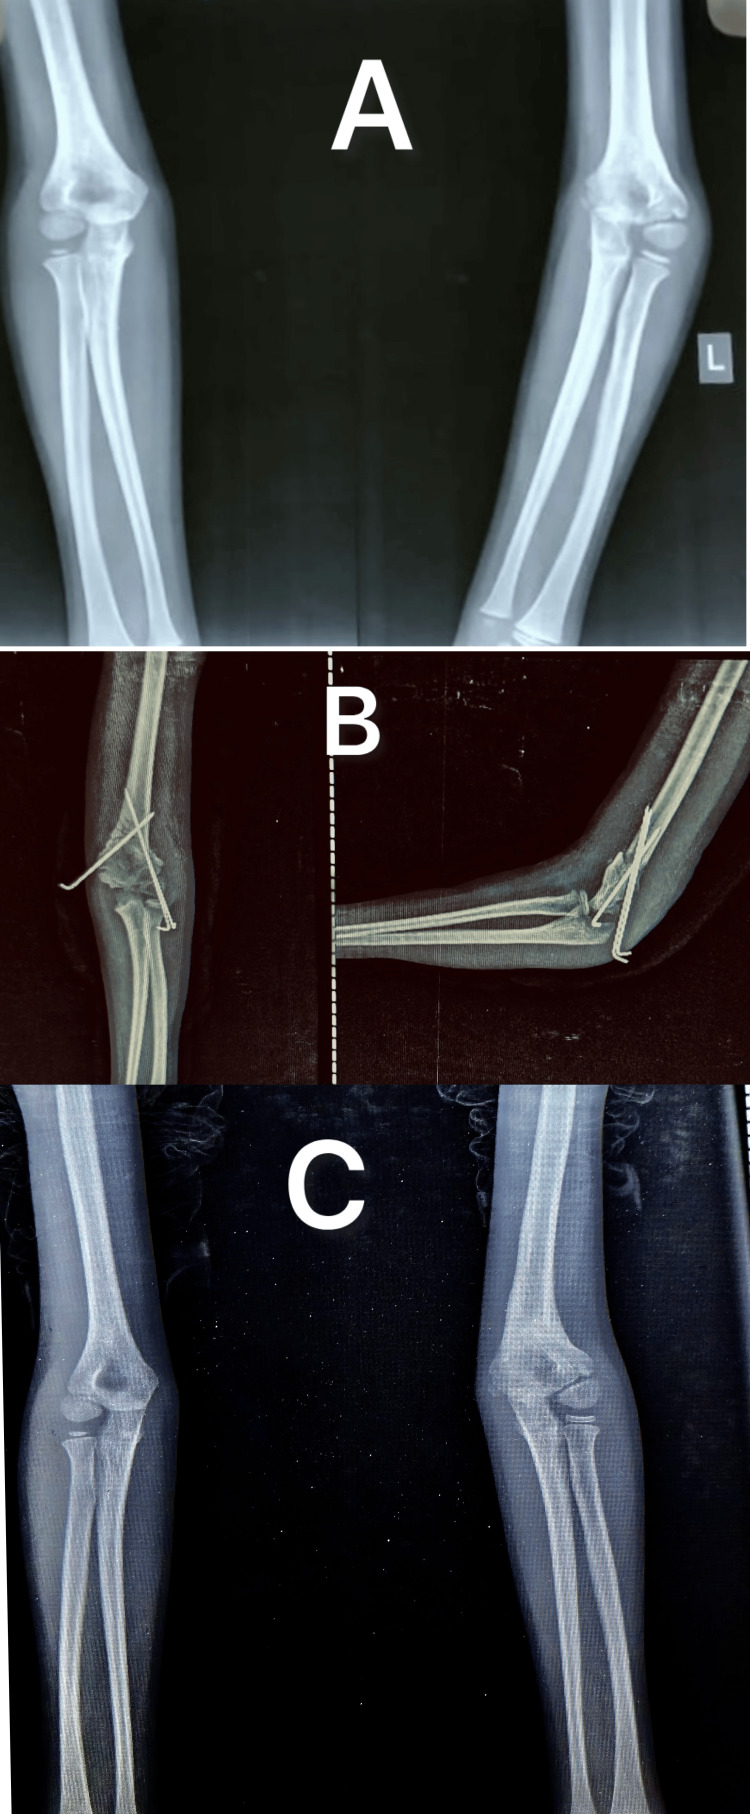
Radiographs showing cubitus varus of the left elbow (A) corrected with a dome osteotomy (B) and achieving proper alignment and healing by three months (C)
